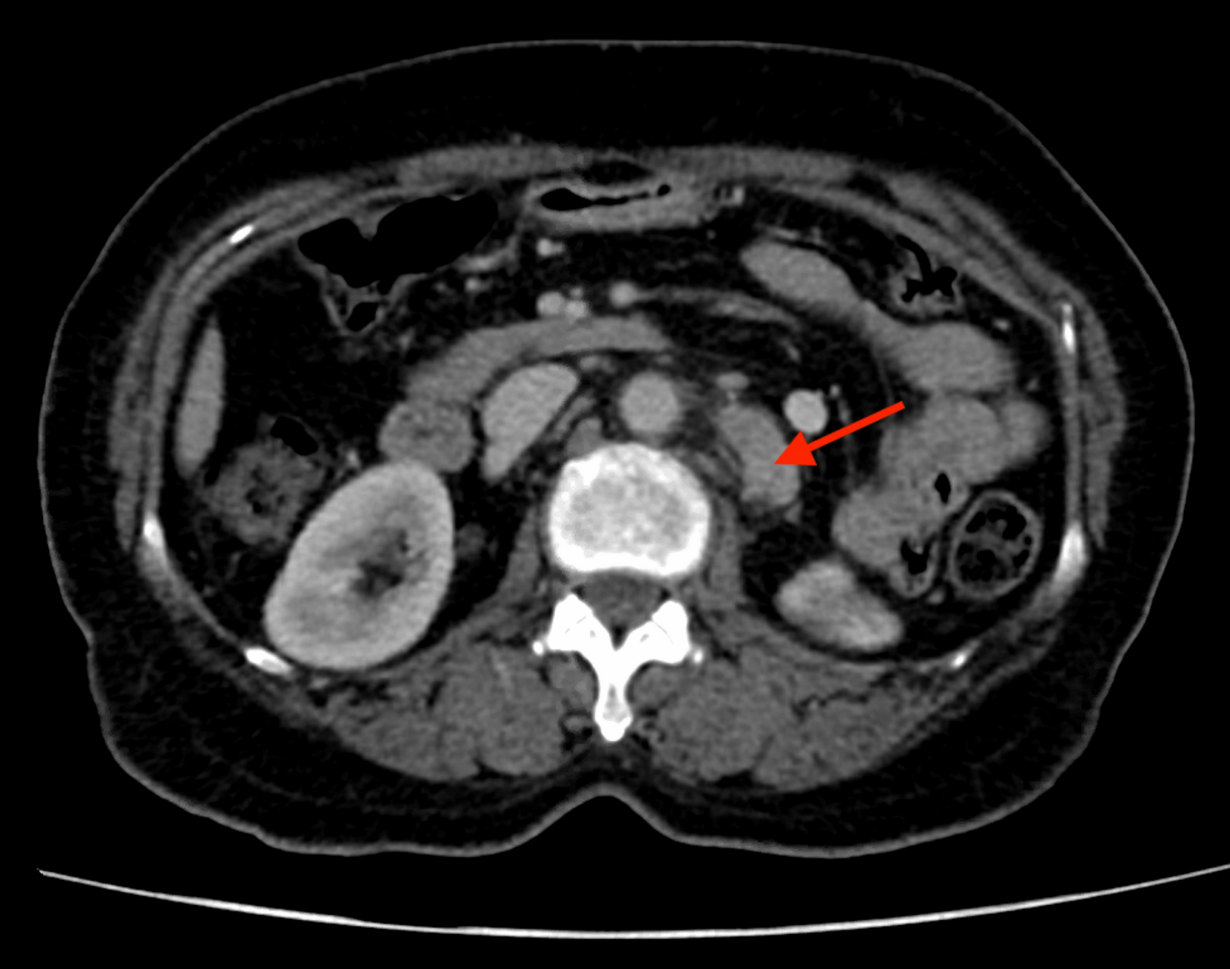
Para-aortic lymphadenopathy on contrast-enhanced computed tomography of the abdomen and pelvis (axial view).
